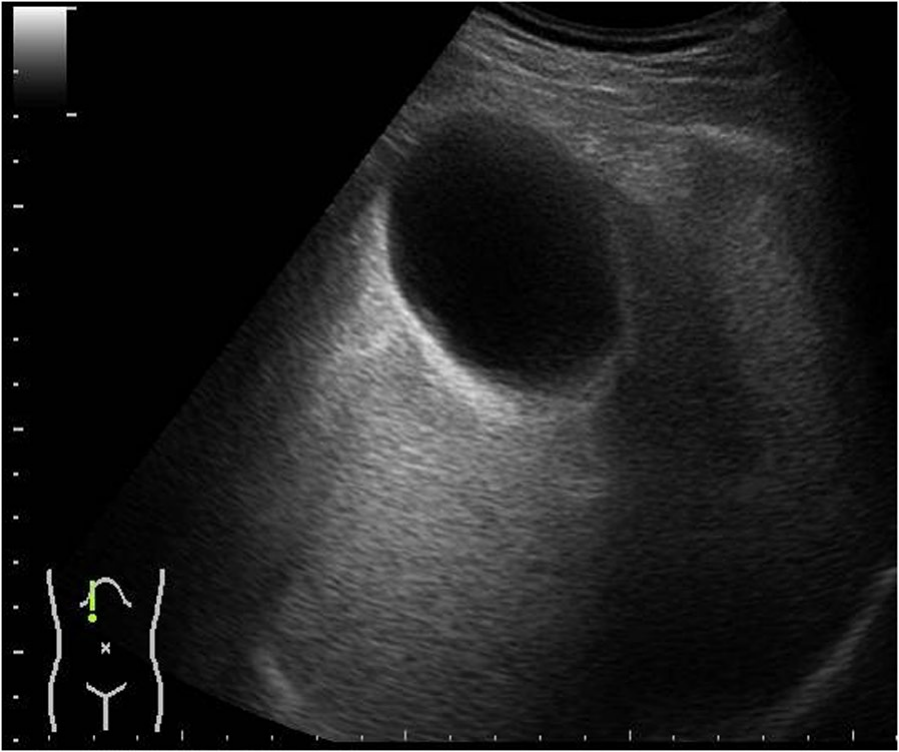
Ultrasound image of the gallbladder
